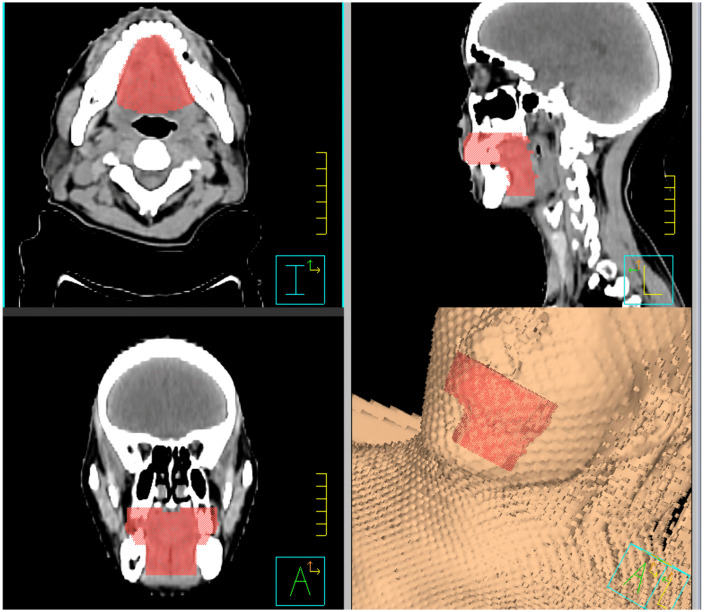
Manual segmentation of the entire oral cavity on CT images from a representative patient.
